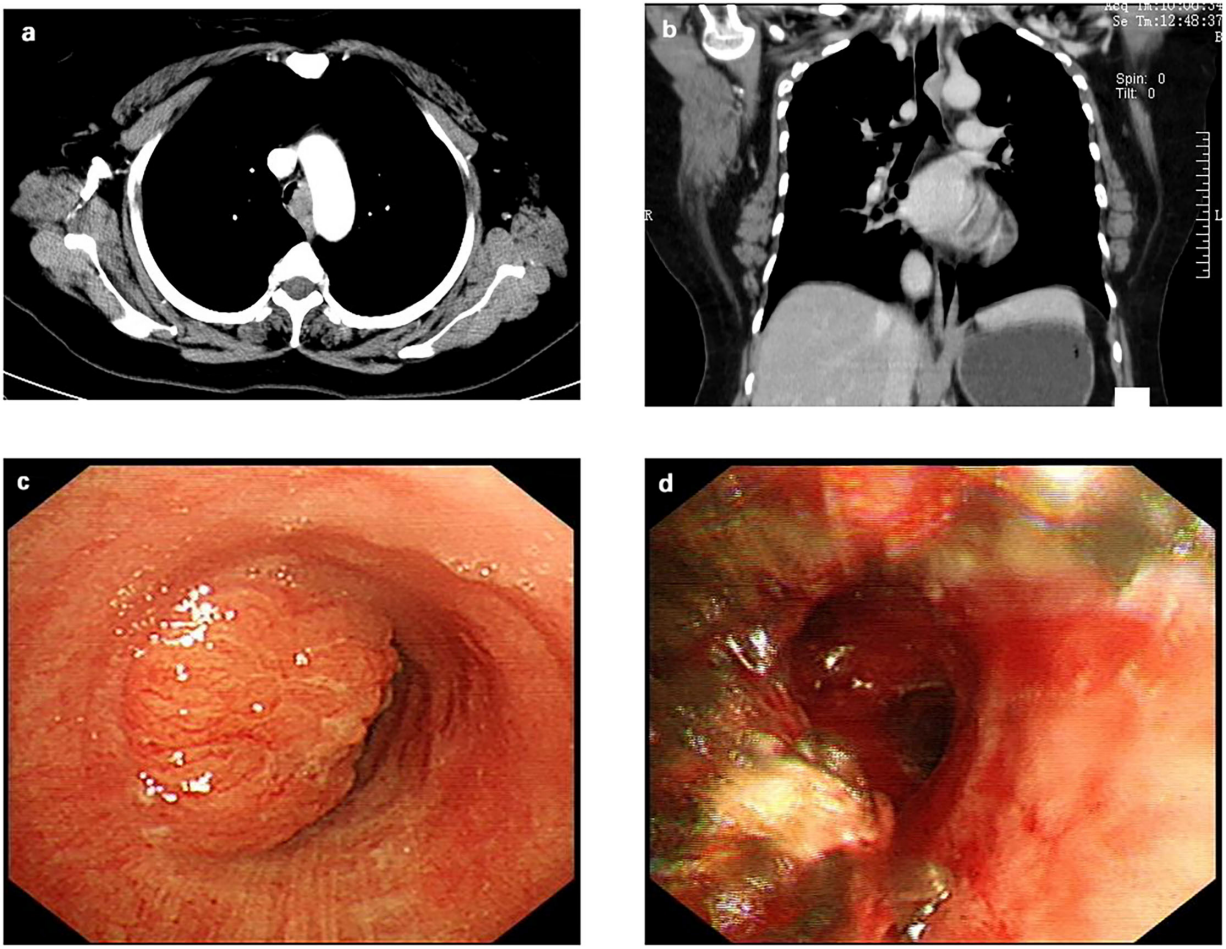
Preoperative examination of the patient. (a) The contrast-enhanced chest computed tomographic scan revealed a tracheal mass occupying a size of ~14 mm × 23 mm located at the T2 and T3 vertebrae; (b) A coronal computed tomographic scan revealed the tracheal mass; (c) A bronchoscopy showed a protruding tumor in the middle and lower trachea with nearly total tracheal occlusion. (d) After a partial tumor was removed via bronchoscopy with argon knife and cryotherapy, the tracheal lumen is more obvious patency than before.
